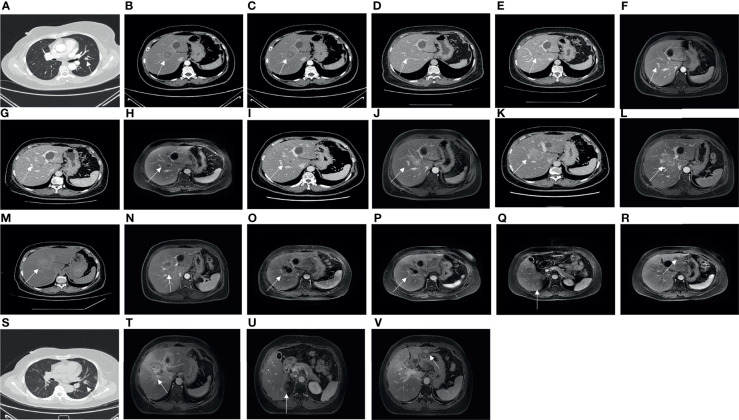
On 2 May 2017, CT showed a pulmonary metastasis with a long diameter of 1.3 cm (A). On 31 May 2017, CT showed a hepatic metastasis with a long diameter of 4.7 cm on the right anterior lobe (B). On 30 October 2017, CT showed a hepatic metastasis with a long diameter of 2.0 cm before involved in a clinical trial of adjuvant endocrine therapy on the right anterior lobe (C). On 30 August 2019, CT showed no apparent hepatic metastasis (D). On 21 November 2019, CT showed a hepatic metastasis with a long diameter of 1.8 cm on the right anterior lobe (unclear boundary) (E). On 25 November 2019, MRI showed a hepatic metastasis with a long diameter of 1.2 cm on the right anterior lobe (F). On 15 June 2020, CT showed a hepatic metastasis with a long diameter of 1.5 cm on the right anterior lobe (G), and MRI showed hepatic metastases, especially the biggest one with a long diameter of 2.7 cm on the right anterior lobe (H). On 11 December 2020, CT showed a hepatic metastasis with a long diameter of 3.2 cm on the right anterior lobe (I), and MRI showed a hepatic metastasis with a long diameter of 3.6 cm on the right anterior lobe (J). On 26 February 2021, CT showed a hepatic metastasis with a long diameter of 3.2 cm on the right anterior lobe (K), and MRI showed a hepatic metastasis with a long diameter of 4.4 cm on the right anterior lobe (L). On 21 April 2021, CT showed a hepatic metastasis with a long diameter of 5.6 cm on the right anterior lobe (M), and MRI showed a hepatic metastasis with a long diameter of 6.0 cm on the right anterior lobe (N). On 6 August 2021, MRI showed a hepatic metastasis with a long diameter of 5.0 cm on the right anterior lobe (O). On 9 October 2021, MRI showed a hepatic metastasis with a long diameter of 3.5 cm on the right anterior lobe (P), a hepatic metastasis with a long diameter of 1.0 cm on the right posterior lobe (Q), and a hepatic metastasis with a long diameter of 1 cm on the left lateral lobe (R). On 21 October 2021, the last CT showed a pulmonary metastasis with a long diameter of 1.0 cm (S). On 15 December 2021, MRI showed a hepatic metastasis with a long diameter of 6.7 cm on the right anterior lobe (T), a hepatic metastasis with a long diameter of 1.0 cm on the right posterior lobe (U), and a hepatic metastasis with a long diameter of 1 cm on the left lateral lobe (V).
